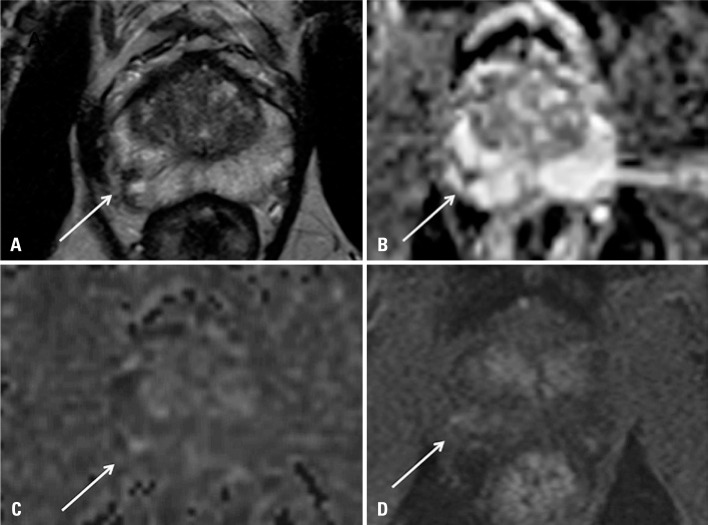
Contrast axial magnetic resonance imaging of the prostate (A) T2-weighted; (B) apparent diffusion coefficient (ADC) map; (C) diffusion sequence and (D) perfusion sequence, showing a small nodule suspected to be a clinically significant malignancy in the periphery of the right lobe (arrows).
